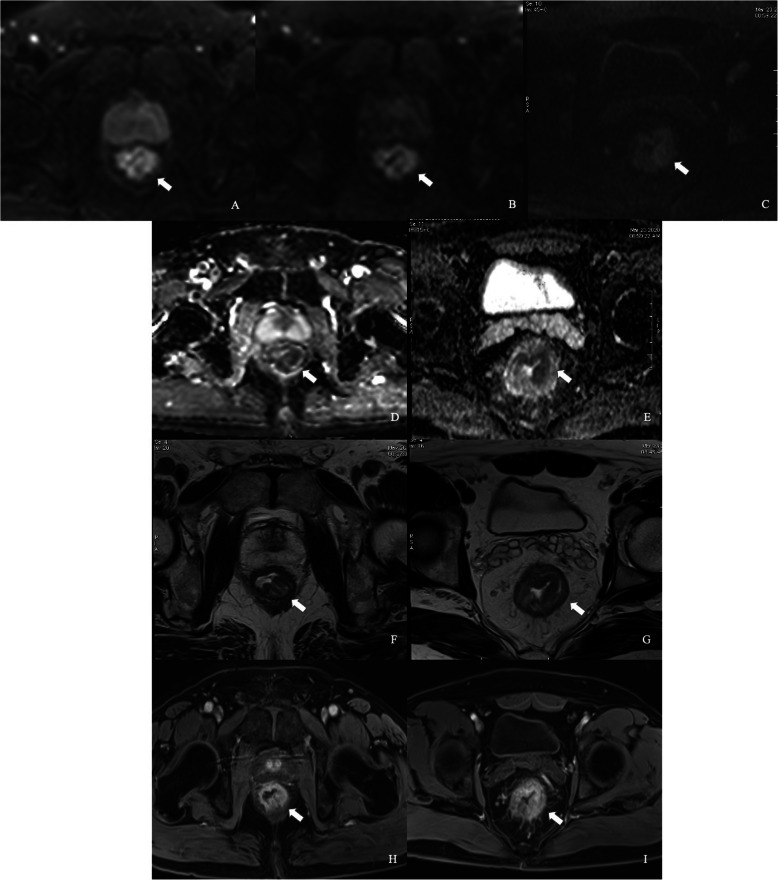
Comparison between iShim- and SS-EPI-DWI in patients with primary rectal cancer. iShim-DWI of b = 800 s/mm^2^ (a) and b = 1600 s/mm^2^ (b) showed higher SNR and CNR with lower signal noise compared with SS-EPI-DWI of b = 1000 s/mm^2^ (c). ADC map (e) of iShim-DWI showed better image quality compared with ADC map (f) of SS-EPI-DWI. T2W images (f and g) and dynamic T1W images (h and i) showed the same location of lesion (arrows) for iShim- and SS-EPI-DWI cohort, respectively
